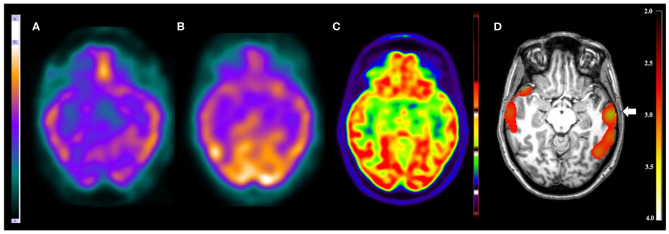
Patient #3 had pharmacoresistant epilepsy. The seizures began at 8 months of age, presenting with 1–2 seizures per week and in some periods 3–5 seizures per day. The EEGs showed epileptiform and non-epileptiform abnormalities in the left temporal region. MRI showed a mild thickening in the opercular region in the left frontal lobe. The reference standard determined the epileptogenic zone to involve the left frontal and left temporal lobes. (A) The ictal and (B) interictal SPECT images visual analysis were inconclusive. The (C)
